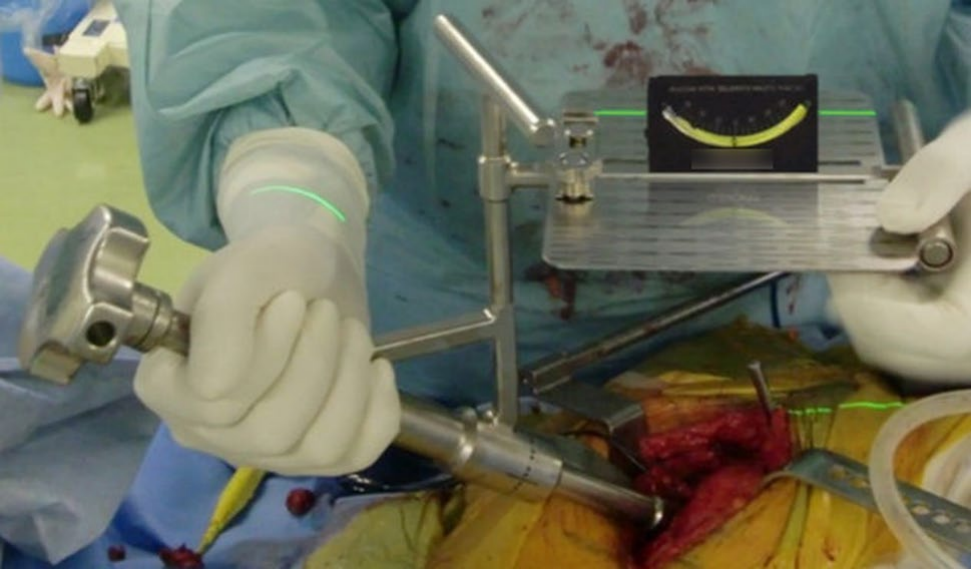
When a green laser beam and a radiographic alignment guide are parallel, and a level gauge indicates 0° in both coronal and axial planes, a cup is supposed to be in 40° of radiographic inclination and 20° of radiographic anteversion.
